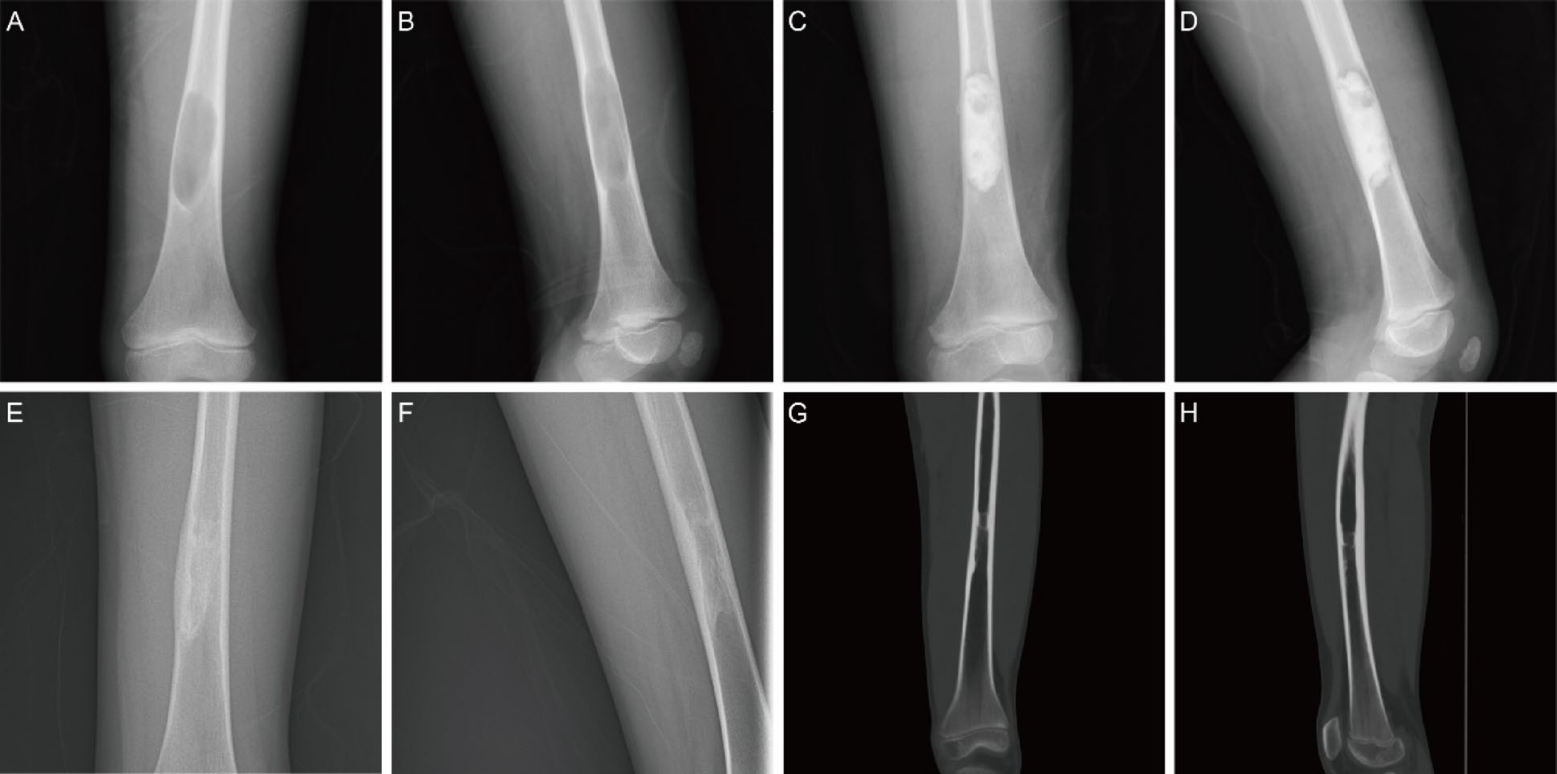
A, B Preoperative X-ray images of the femur UBC in a 6-year-old boy. C, D Postoperative X-ray images on the day after surgery. E, F Postoperative X-ray images at 28 months of follow-up. G, H Postoperative CT images at 73 months of follow-up showed complete radiographic healing
